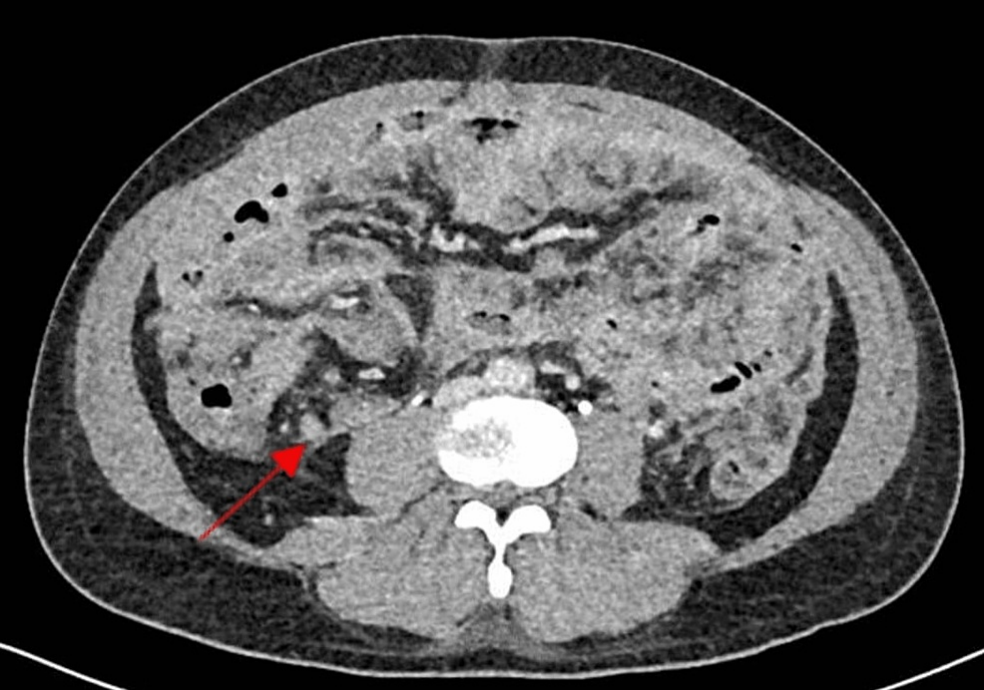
Computed tomography scan showing distal ileum bowel wall thickening
